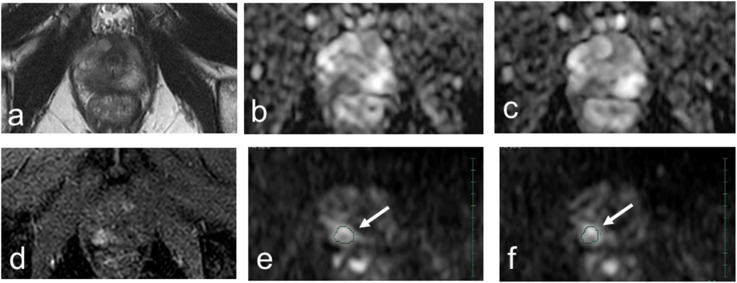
Multiparametric magnetic resonance imaging of the case (70 years, right peripheral zone cancer, GG of 3, PI-RADS of 4, T2aN0M0). a Axial T2-weighted image (repetition time of 4,000 ms and echo time of 80 ms). b First axial apparent diffusion coefficient (ADC) map (100, 1,000). c Second axial ADC map (100, 1,000). d Dynamic contrast-enhanced T1-weighted image. e First DWI 1,500. f Second DWI 1,500. Arrows indicate polygonal areas of the region of interests on e and f
